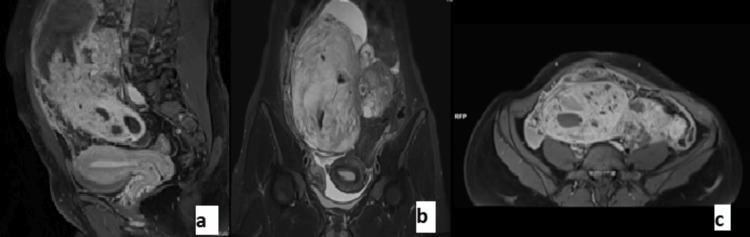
Contrast-enhanced MRI Contrast-enhanced MRI showing multilobulated multicystic lesion of abdomen and pelvis in (a) sagittal view, (b) coronal view, and (c) transverse sectional view.
